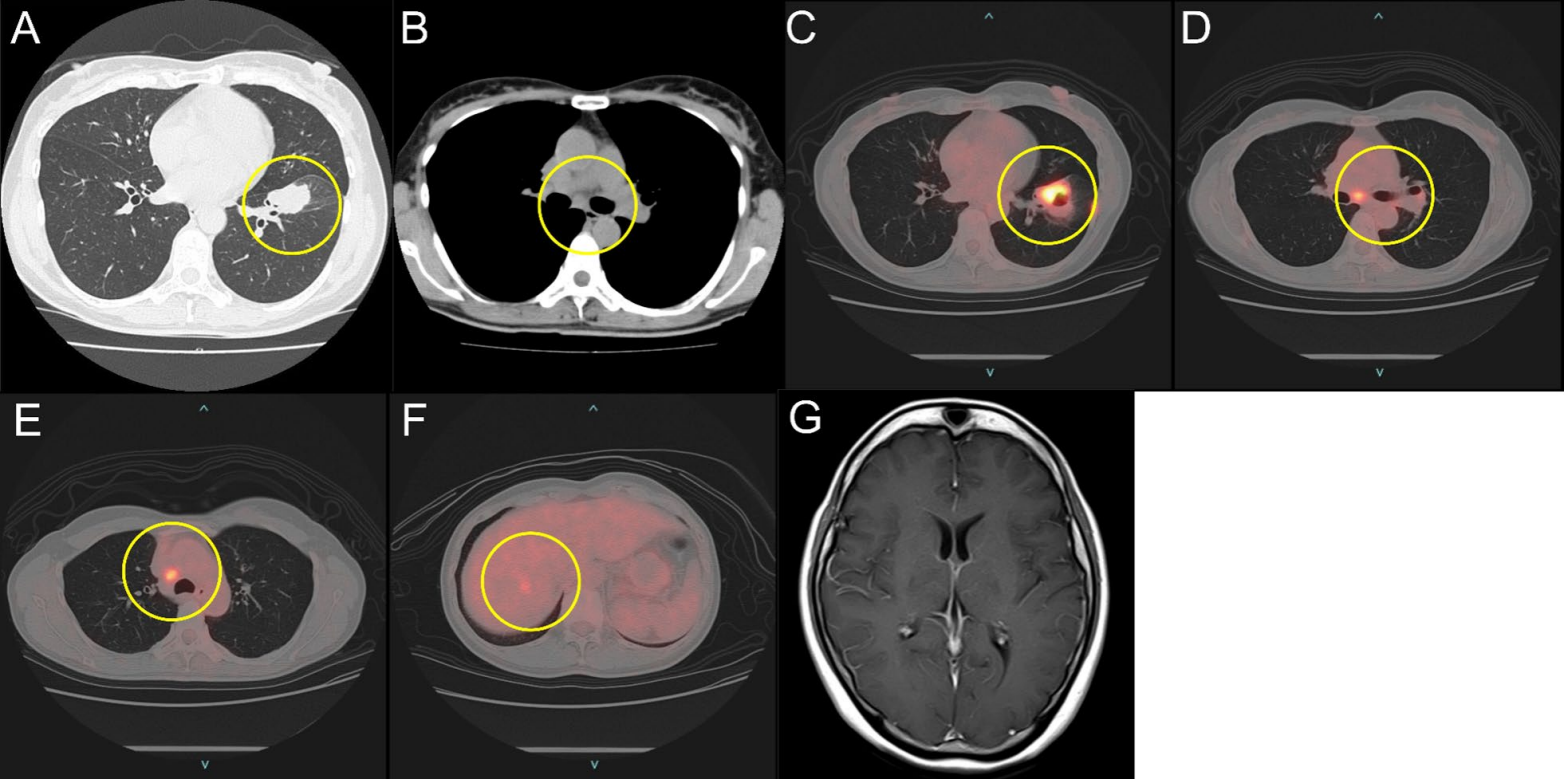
CT images showing A 36-mm tumor in left lower lobe and B swelling of the subcarinal lymph node. PET-CT revealed C a high level of accumulation of the primary tumor (SUVmax 10.5), as well as metastases in the D subcarinal lymph node, E right mediastinal lymph nodes, and F liver. G Contrast enhanced brain MRI findings showed no brain metastasis
